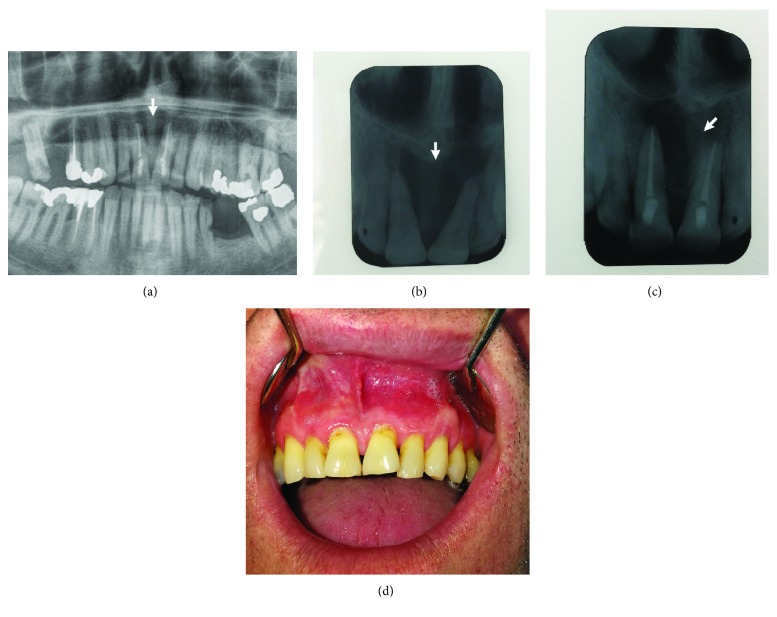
Follow-up period one 1 year after surgery. (a) OPG with no signs of radiolucency. (b) Preoperative X-ray with obvious signs of radiolucency. (c) Postoperative X-ray one year after surgery with signs of bone regeneration in progress. (d) Clinical appearance of vestibular mucosa one year after surgery (no swelling, no inflammation).
